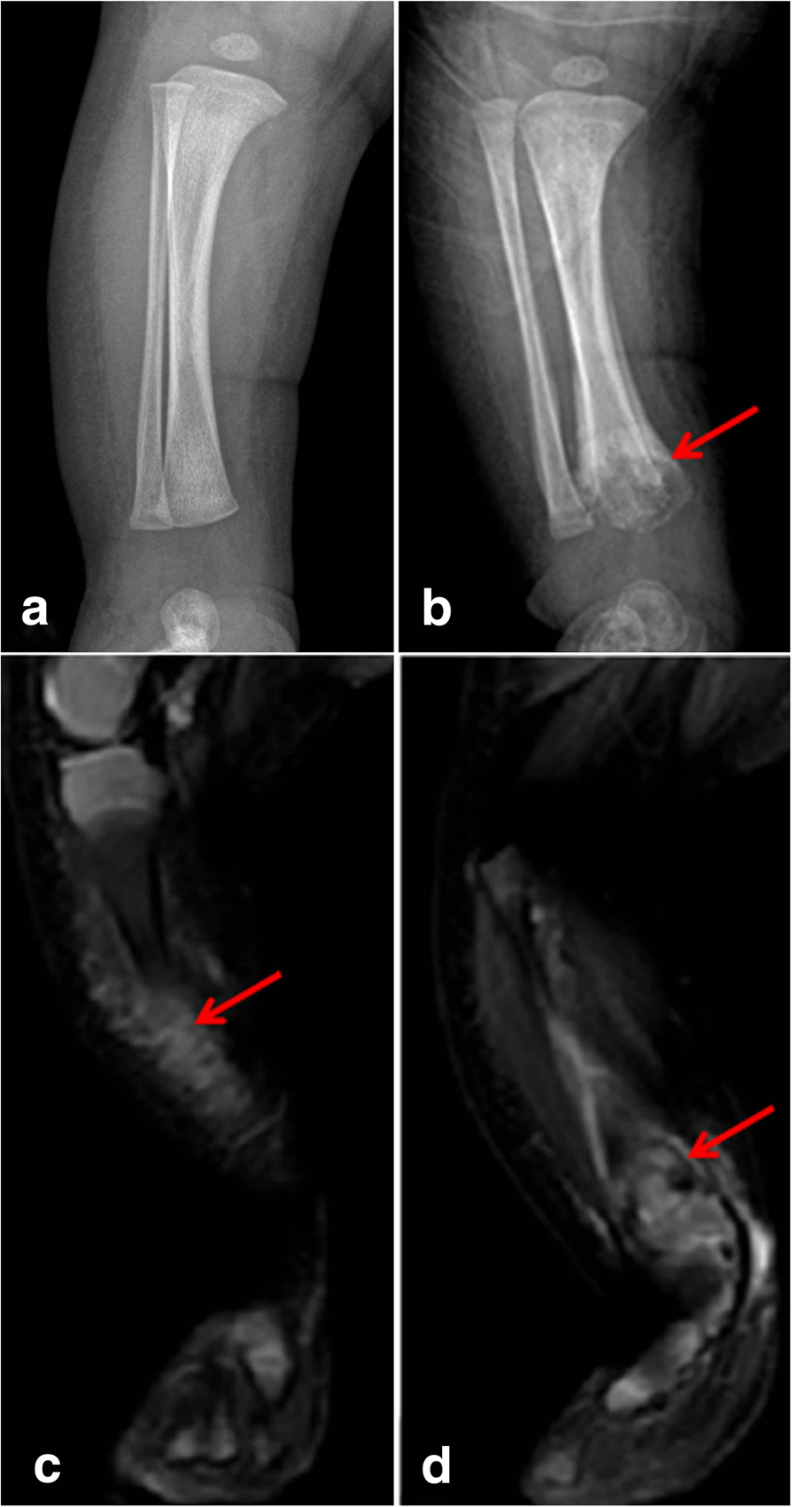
The radiography of the right lower limb on admission (a) compared with the radiography of the same limb at diagnosis (b) shows the destruction of the distal tibia, and the periosteal reaction (red arrow). The MRI of the right lower limb shows significant edema of the soft tissue (c), the destruction of the distal tibia and the periosteal reaction of the tibia and fibula (d) (red arrow)
